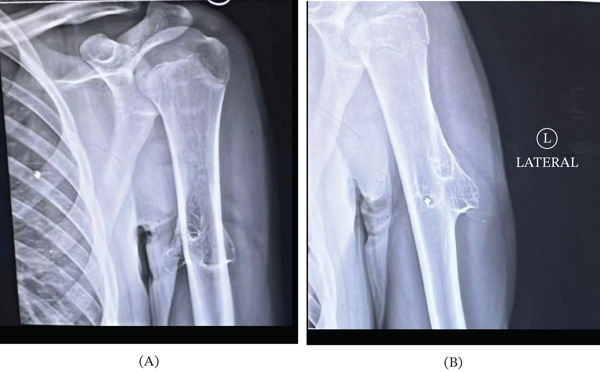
Plain radiographs of the left humerus demonstrating a multilobed osteochondroma arising from the posterior humeral midshaft. (A) Anteroposterior (AP) projection showing a lobulated osseous exostosis at the humeral midshaft with demonstrable continuity of the lesion with the underlying medullary cavity and native cortex. (B) Lateral projection demonstrating the posterior location of the lesion and its pedunculated morphology, with no evidence of cortical breach or aggressive periosteal reaction.
